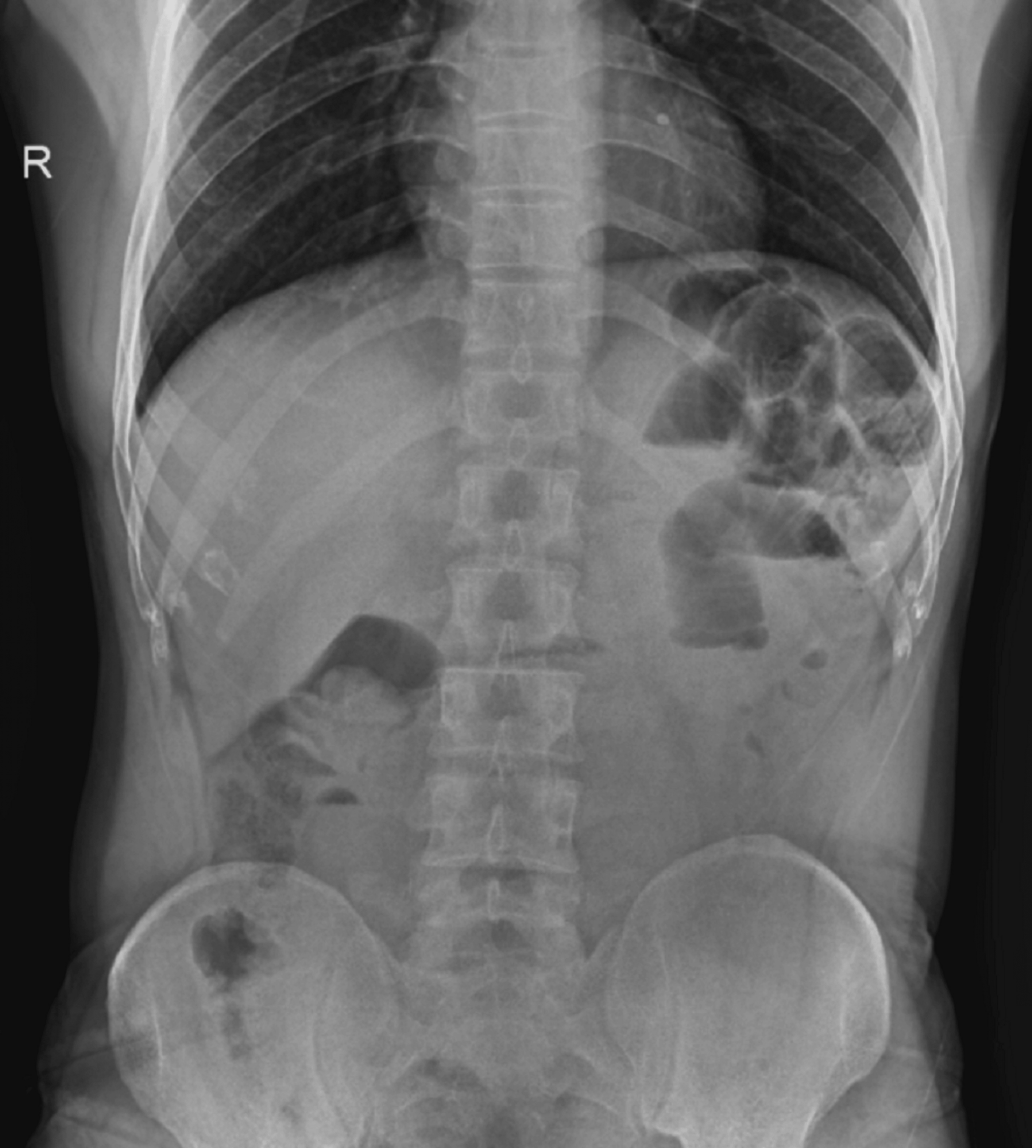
Erect X-ray of the abdomen showing multiple air fluid levels suggesting small bowel obstruction.
